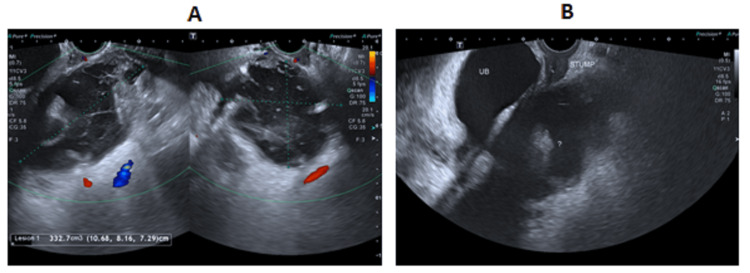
Ultrasound images (first ED visit) (A) Large heterogeneous pelvic collection (hematoma) showing septations and internal echoes. (B) Post-hysterectomy status with the vaginal stump. ED, emergency department
